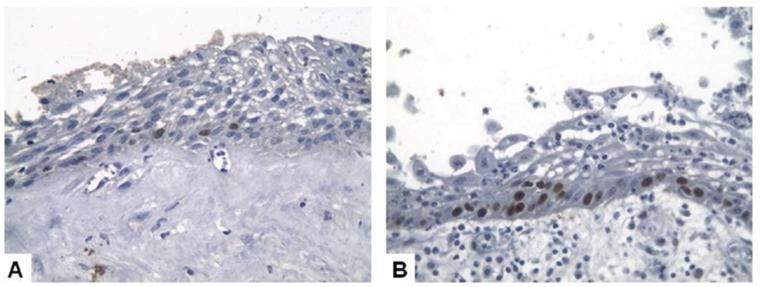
Ki-67 immunoexpression in basal and immediately suprabasal cells from the epithelial lining in a periapical cyst (A) and a residual cyst (B) (A and B - Immunoperoxidase, 40x).
